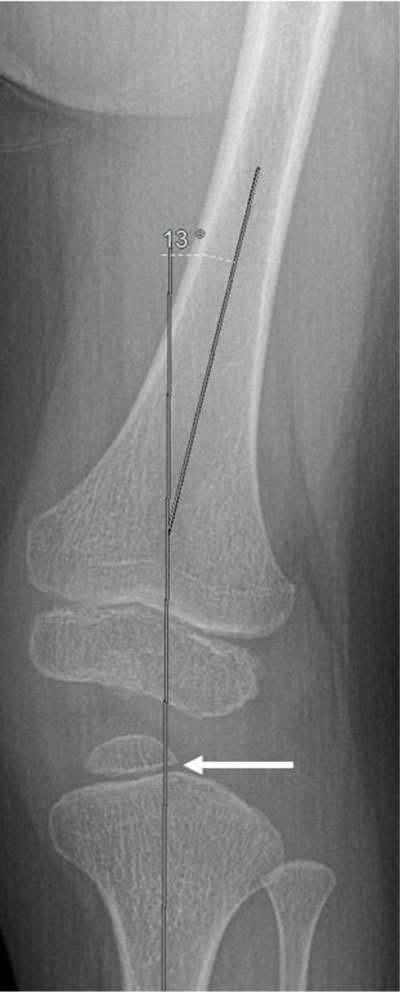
Anteroposterior radiograph of the left knee shows genu valgum (13°) and hypoplasia of the lateral proximal epiphysis of the tibia (arrow).
